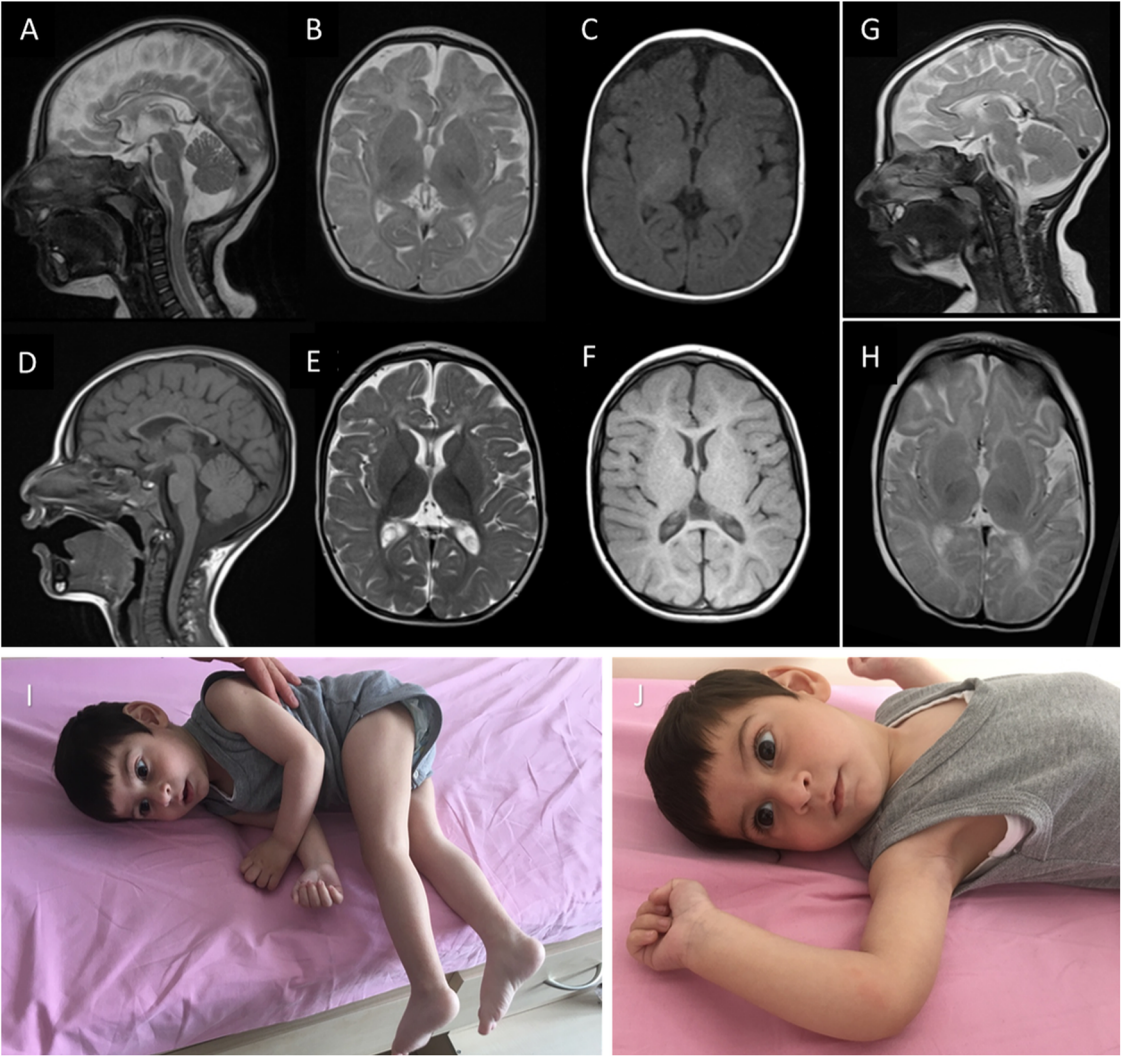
MRI findings and phenotypes of our patients with ASNSD. Brain MRI of patient 1 obtained at 4 months old (upper row, a–c) and 3.5 years old (lower row, d–f). Delayed myelination is best appreciated on the T1-weighted axial image obtained at 4 months old (c) and on the T2-weighted axial images obtained at 3.5 years old (e). The thin corpus callosum is displayed well in both sagittal T2- and T1-weighted images (a+d), while cerebral atrophy and the simplified gyration prominent in the frontal lobes were found on follow-up imaging (d–f). Brain MRI of patient 2 obtained at 4 months of age (g+h). The sagittal T2-weighted image (g) shows a very thin corpus callosum. Delayed myelination is better appreciated in image (h). The clinical phenotype of patient 2 (i+j) included absent fixation, reduced attention to external stimuli, and central hypotonia, which made him unable to sit without support
